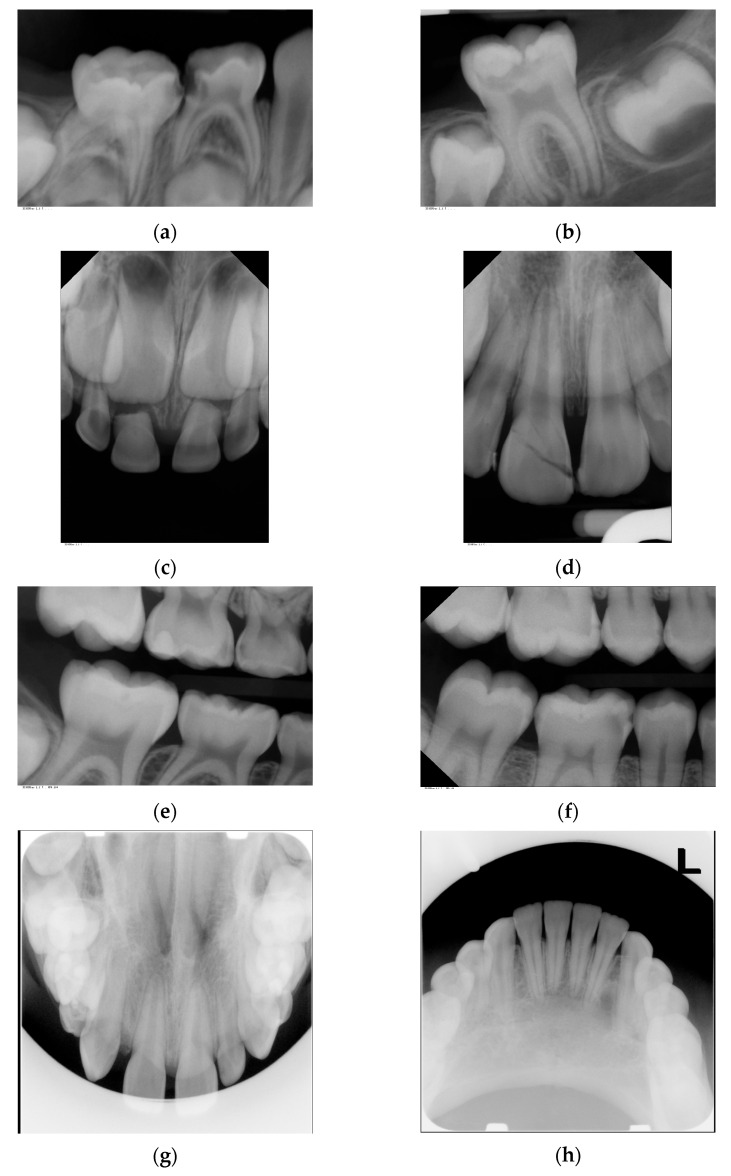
Examples of investigated intraoral radiographs: (a) dental radiograph of primary molars for caries and apical diagnostics; (b) dental radiograph of permanent molar for caries progression and apical diagnostics; (c) dental radiograph of primary teeth for trauma diagnostics; (d) dental radiograph of permanent teeth for trauma diagnostics; (e) bitewing radiograph for caries detection in primary molars; (f) bitewing radiographs for caries diagnostics in permanent molars; (g) occlusal radiograph for diagnosis of tooth germ position in the upper jaw; (h) occlusal radiograph for diagnosis of pathology in the lower jaw.
